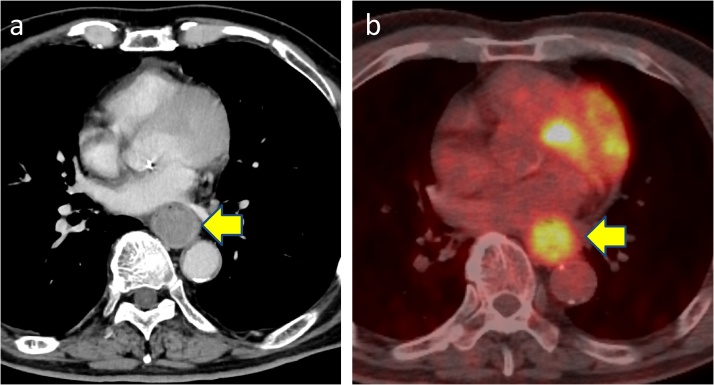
a) CT scan showed large mass in the lower esophagus. b) PET-CT showed a hypermetabolic esophageal tumor (SUV_max_ = 7.89).
